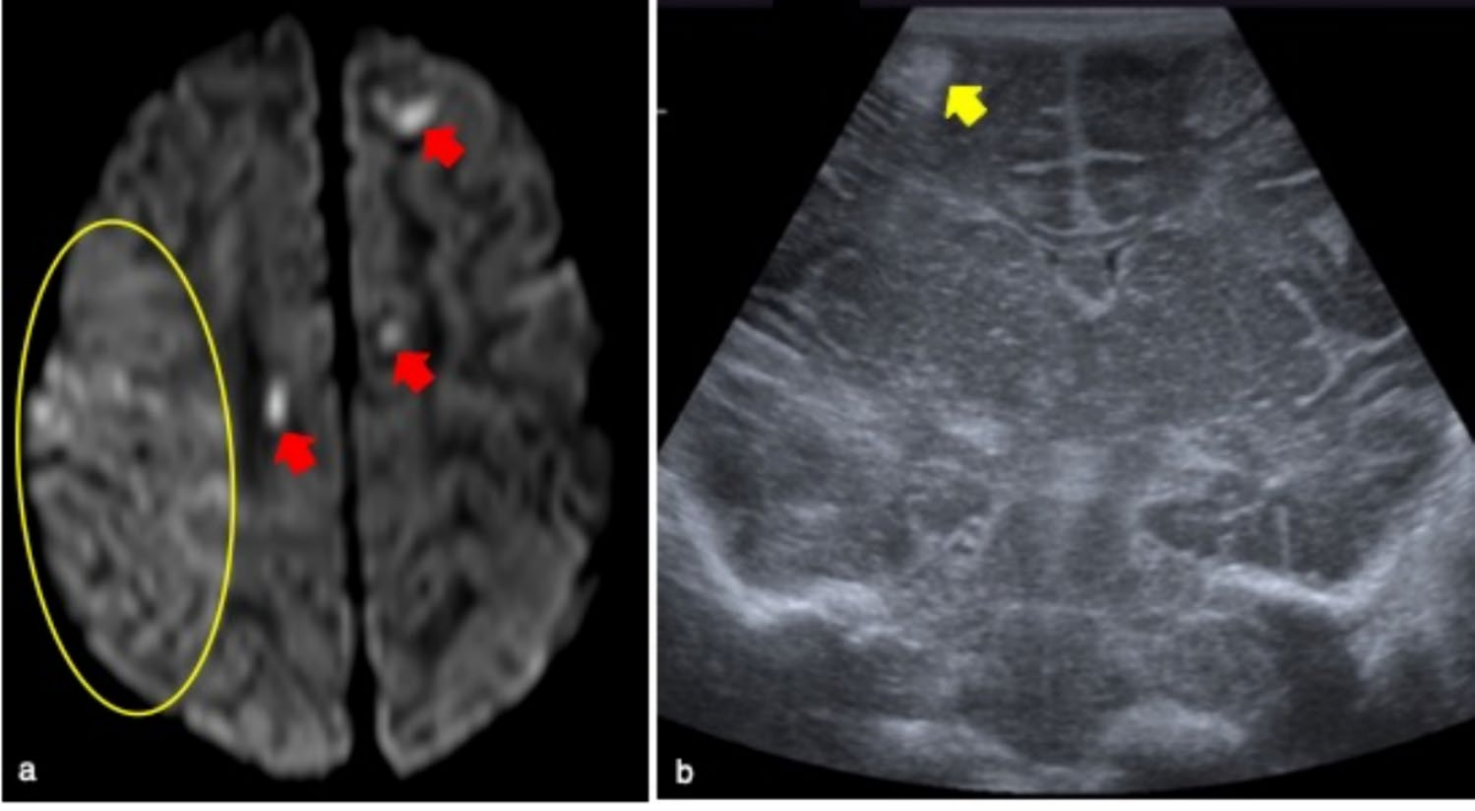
Full term female infant who underwent cerebral ultrasound at 3-days of life for central apneas and epileptic seizures. No cerebral pathological findings were identified on first ultrasound evaluation (not showed), therefore MR was performed at day 4 of life to detect brain anomalies. Right-side acute ischaemia of the temporal lobe and multiple cortical petechiae were detected on DWI (yellow circle and red arrows in a, respectively), which represent typical findings of hypoxic-ischemic encephalopathy. Follow-up cerebral ultrasound showed a corticalfocal hyperechoic area on coronal view as outcome of ischaemia (yellow arrow in b)
